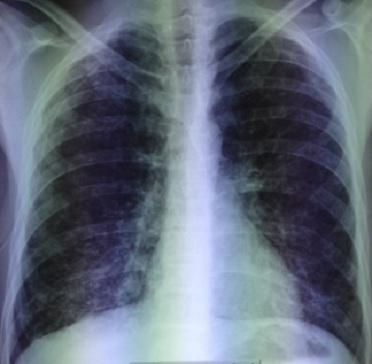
Radiographie thoracique après drainage montrant des lésions kystiques bilatérales
